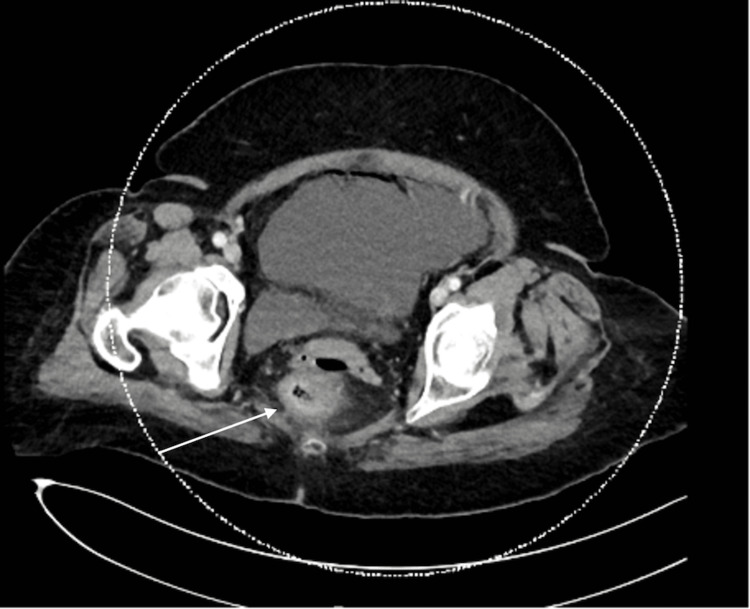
Abdominopelvic CT scan showing diffuse rectal mural enhancement (white arrow) and thickening with mesorectal smudging.
